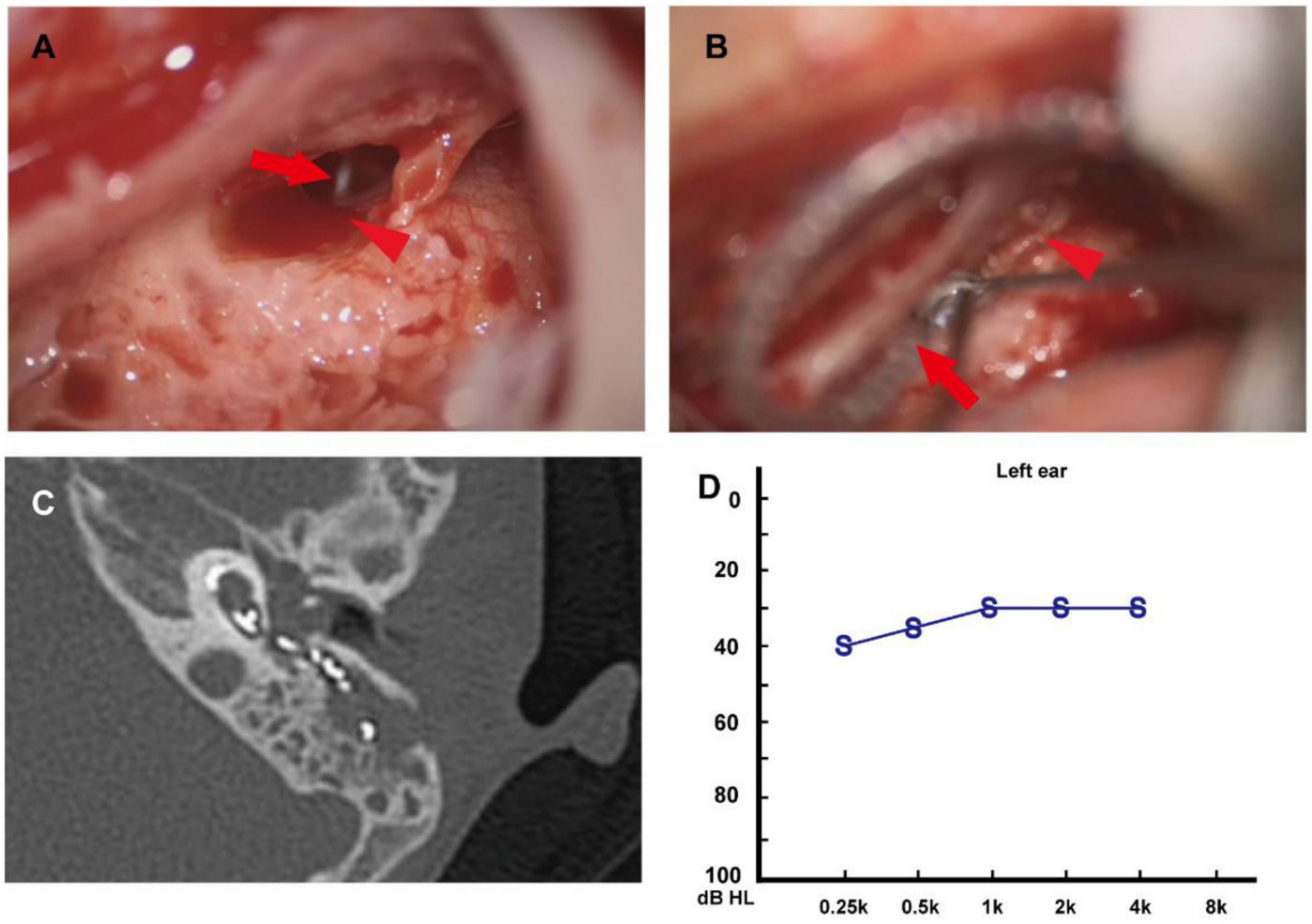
(A) Intraoperatively, the facial recess was opened. The red arrowhead indicates clear cerebrospinal fluid leaking around the oval window. The red arrow points to the stapedius muscle. (B) The cochlear electrode was implanted via the round window. The red arrowhead denotes the cessation of cerebrospinal fluid leakage after the oval window area was obliterated with muscle. The red arrow indicates the cochlear electrode. (C) Postoperative CT demonstrates correct intrascalar placement of the cochlear implant electrode. (D) The one-month postoperative sound field audiometry demonstrates the hearing results of the left ear.
